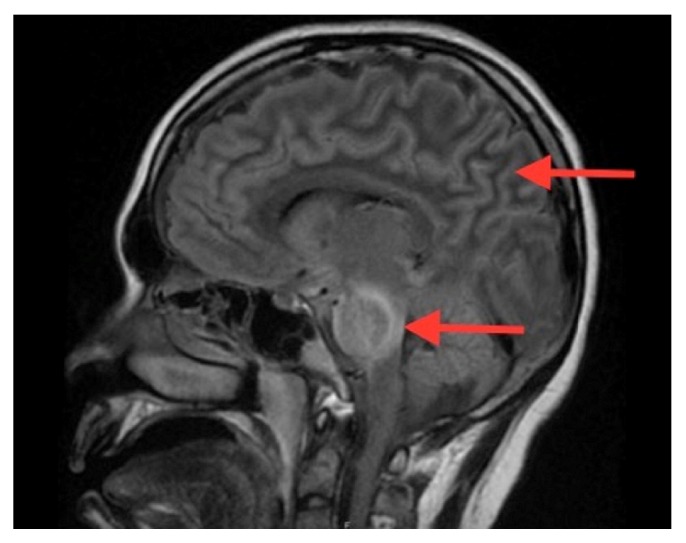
T2-FLAIR Sagittal image showing diffuse pontine edema and cerebral edema.
